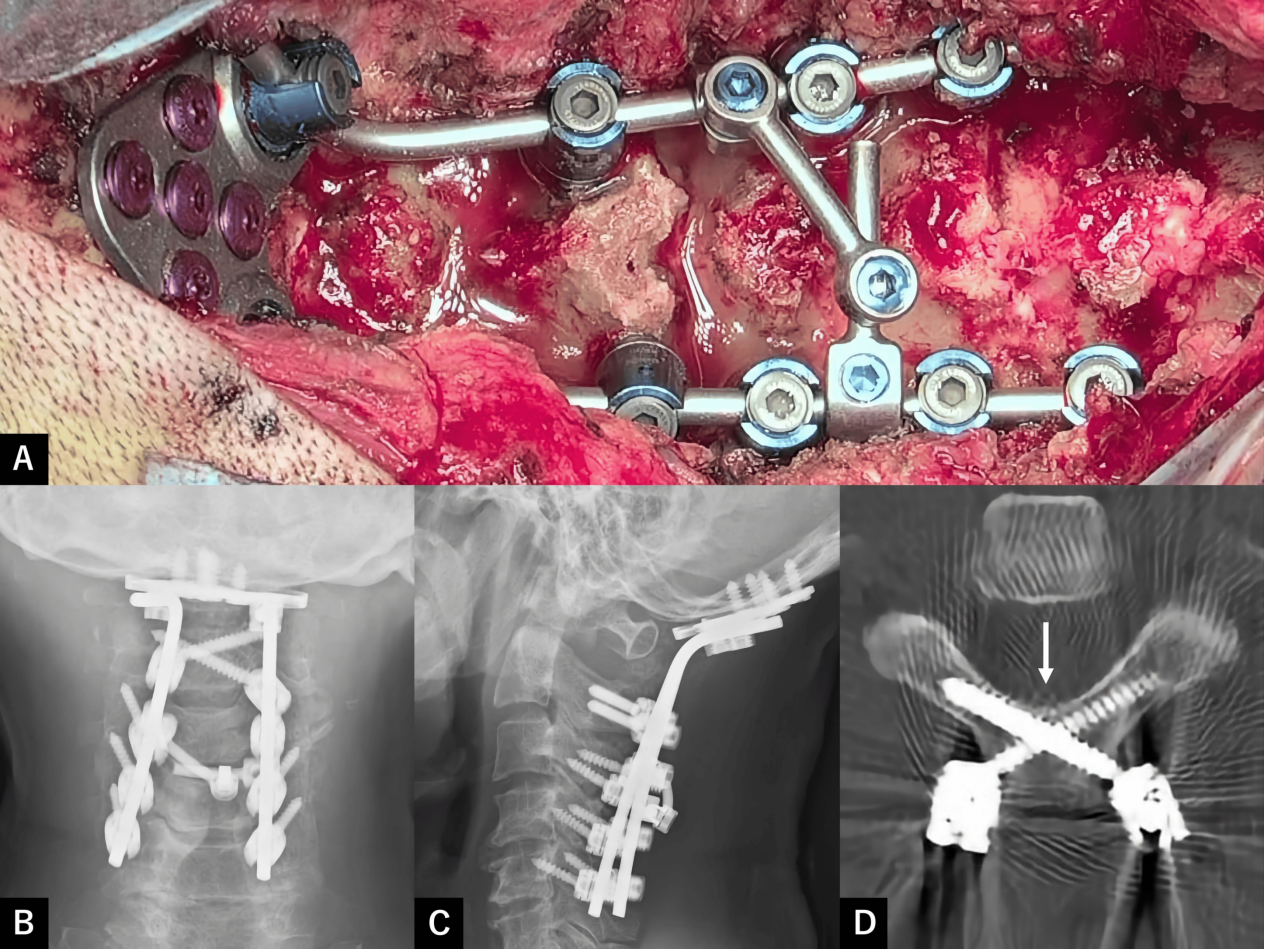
Intraoperative photograph and imaging findings at one month after surgery (A) Intraoperative photograph. (B) Anteroposterior cervical X-ray. (C) Lateral cervical X-ray. (D) Axial section of cervical CT. We performed occipito-C2-C5 posterior fixation, which included the insertion of C2 translaminar screws and C3-C5 lateral mass screws. One month after surgery, imaging studies confirmed the stability of the fixed screws and rods. Cervical CT showing bilateral C2 translaminar screws (arrow).
